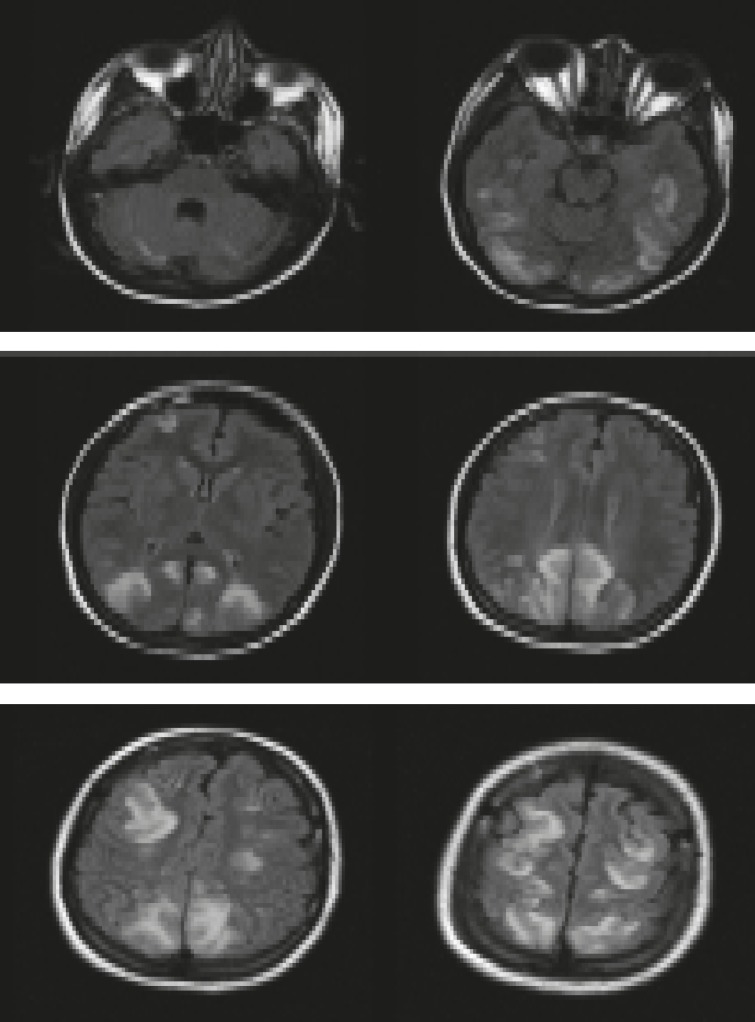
Cerebral MRI in a patient with PRES
